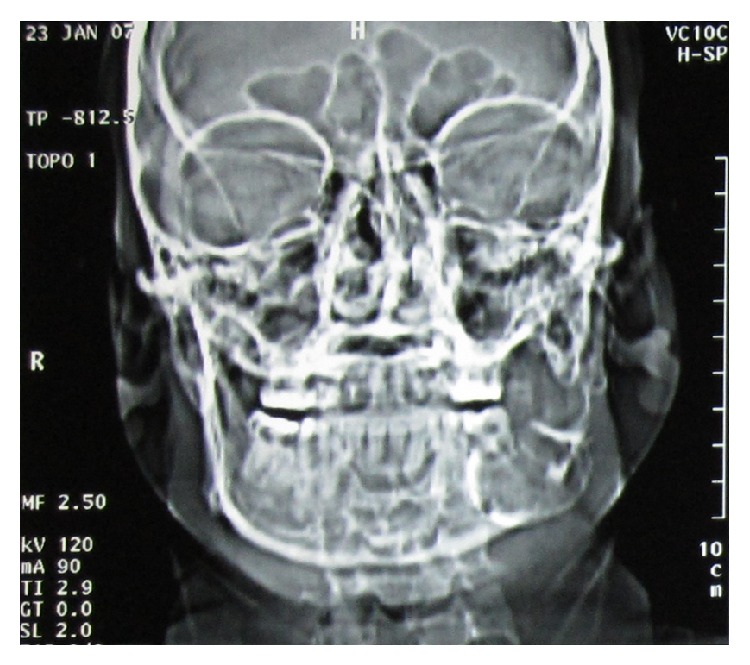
Coronal section of CT with visible buccal bone expansion.
